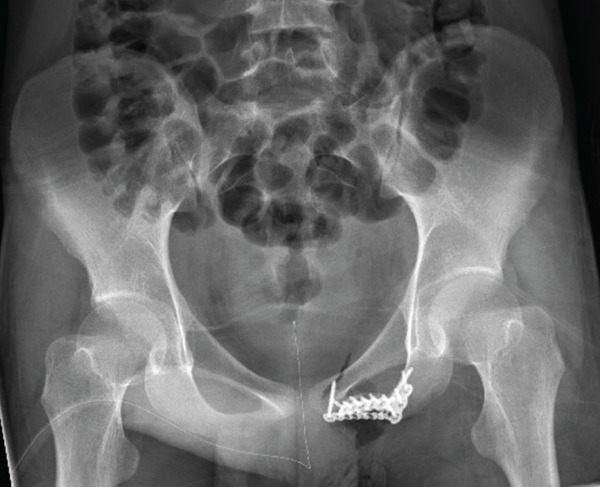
Anteroposterior (AP) pelvis radiograph demonstrating fixation of the inferior pubic ramus osteotomy fragment.
